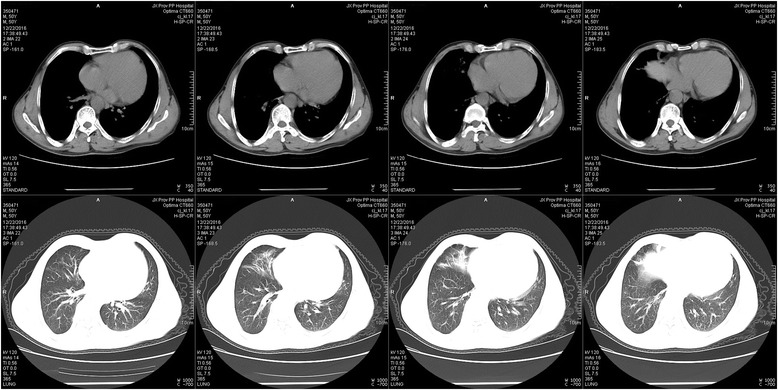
The third Chest CT (Dec. 22) showed the shadow had basically resolved, with a residual fibre cable
